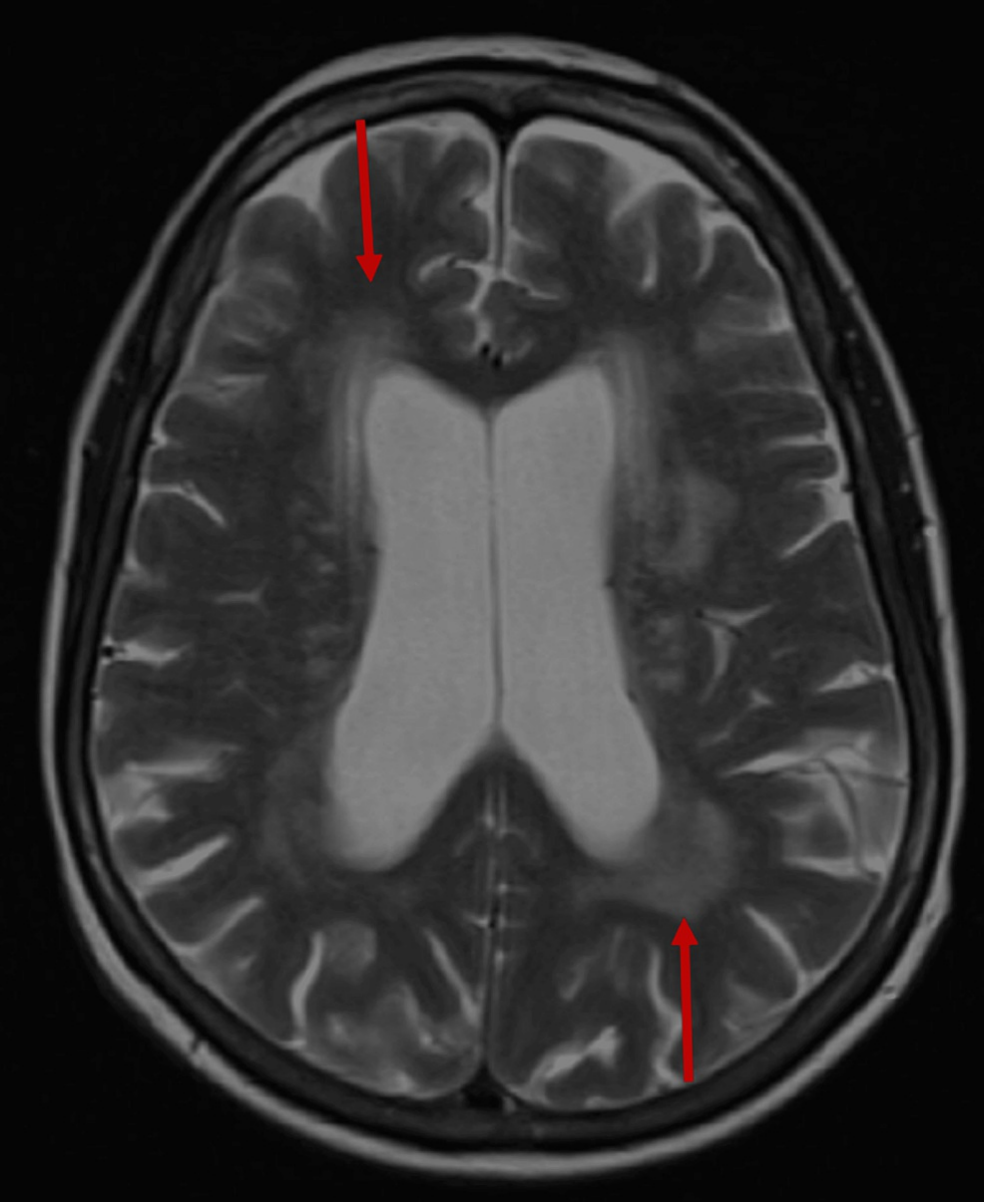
T2-weighted MRI demonstrating enlargement of the lateral ventricles. There is also confluent periventricular hyperintensity representing transependymal CSF flow (red arrows) in the setting of normal pressure hydrocephalus.
